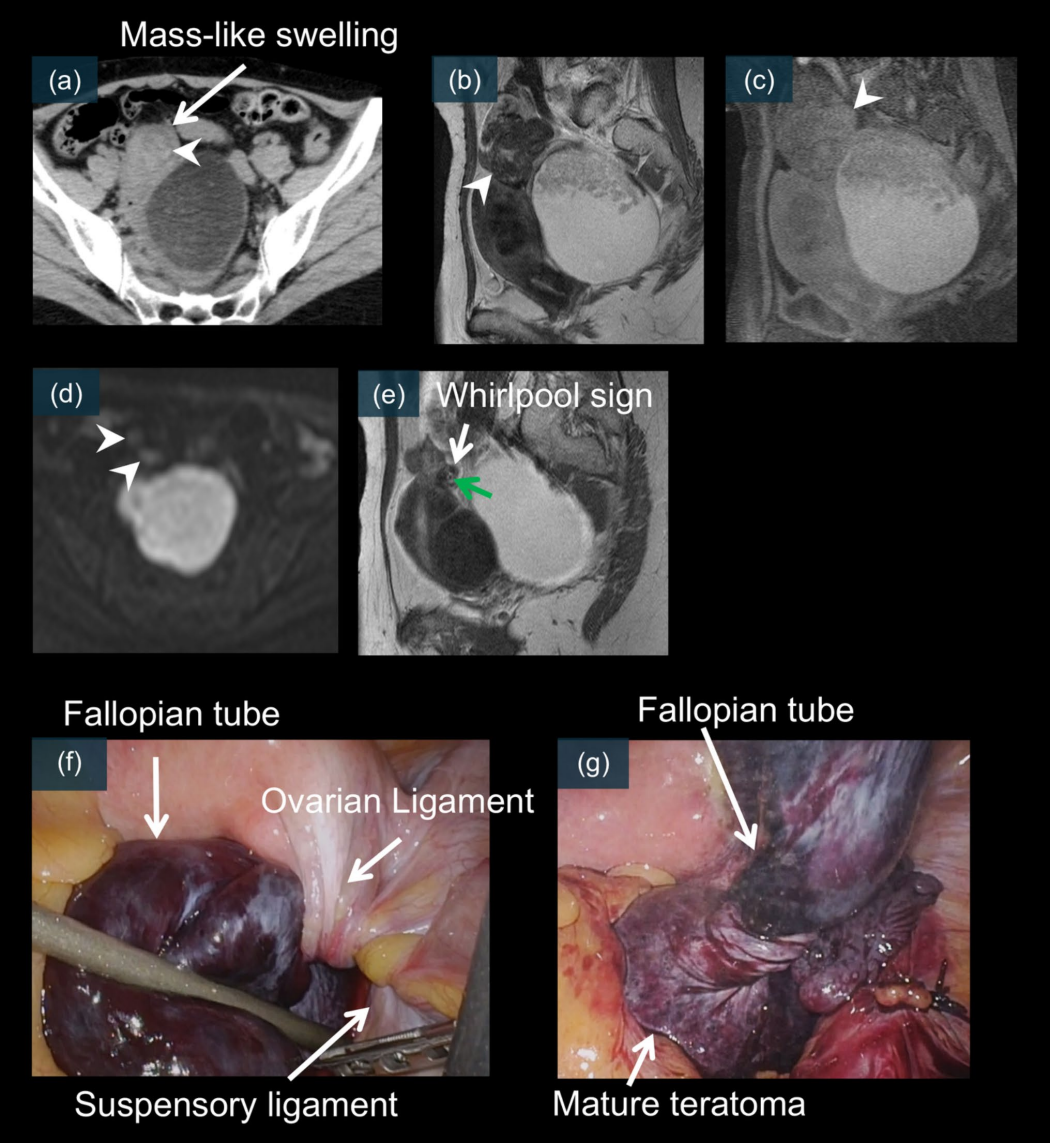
A 55-year-old female. a is an unenhanced CT image. Although a clear whirlpool sign is not evident, there is a mass-like structure adjacent to the mature cystic teratoma (white arrow). This is referred to as a “mass-like swelling,” indicating the presence of an enlarged fallopian tube and mesosalpinx containing dilated veins between the uterus and the twisted ovary. Heterogeneous high-density areas are visible within it (a, white arrowhead), which also show low signal intensity on T2WI (b, white arrowhead), high signal intensity on fat-suppressed T1WI (c, white arrowhead), and DWI (d, white arrowhead), likely corresponding to hemorrhage into the interstitial tissue. e shows a clear whirlpool sign (white arrow), with a flow void (green arrow) in the center thought to be the ovarian artery, suggesting a twisted vascular pedicle. f and g are laparoscopic images, which reveal a 720° torsion of the right mature cystic teratoma and fallopian tube
